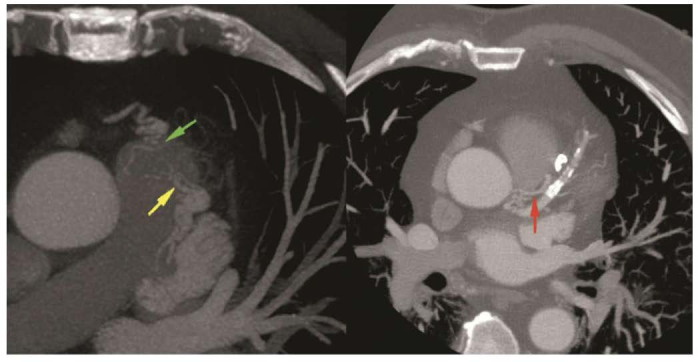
Cardiac computed tomography angiography images revealing: CAF
